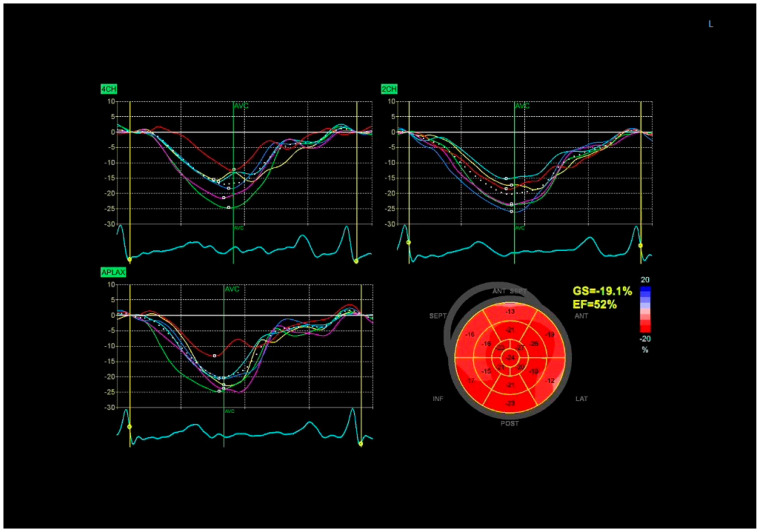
Global longitudinal strain (GLS) in normal left ventricular systolic function. Speckle-tracking echocardiography from apical views shows uniform strain curves with preserved segmental deformation. The bull’s-eye plot demonstrates normal GLS (−19.1%) and ejection fraction (52%).
